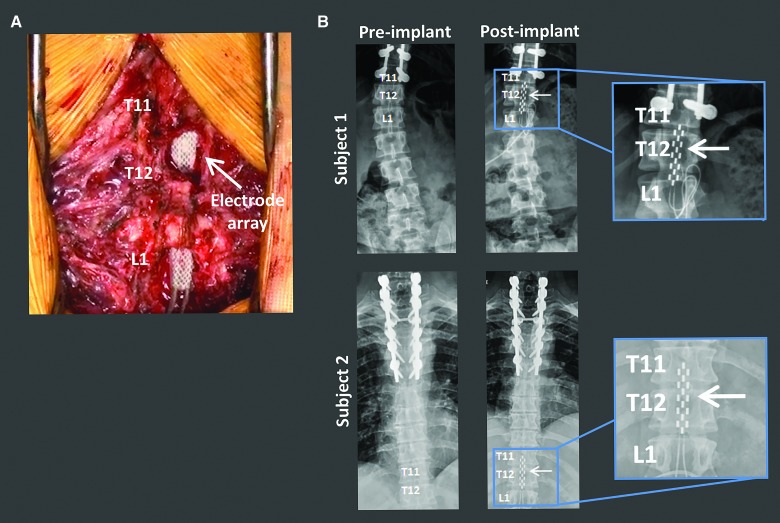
Surgical implantation of the EES electrode array spanning the lumbosacral spinal cord. (A) Intraoperative image of the location of the EES array at the T11–L1 vertebral levels. (B) Anterior-posterior X-ray of each subject before and after EES electrode array implantation. Subject 1 imaging was captured in a seated position. Subject 2 imaging was captured while lying supine. Inserts depict zoomed in view of the EES array. EES, epidural electrical stimulation.
